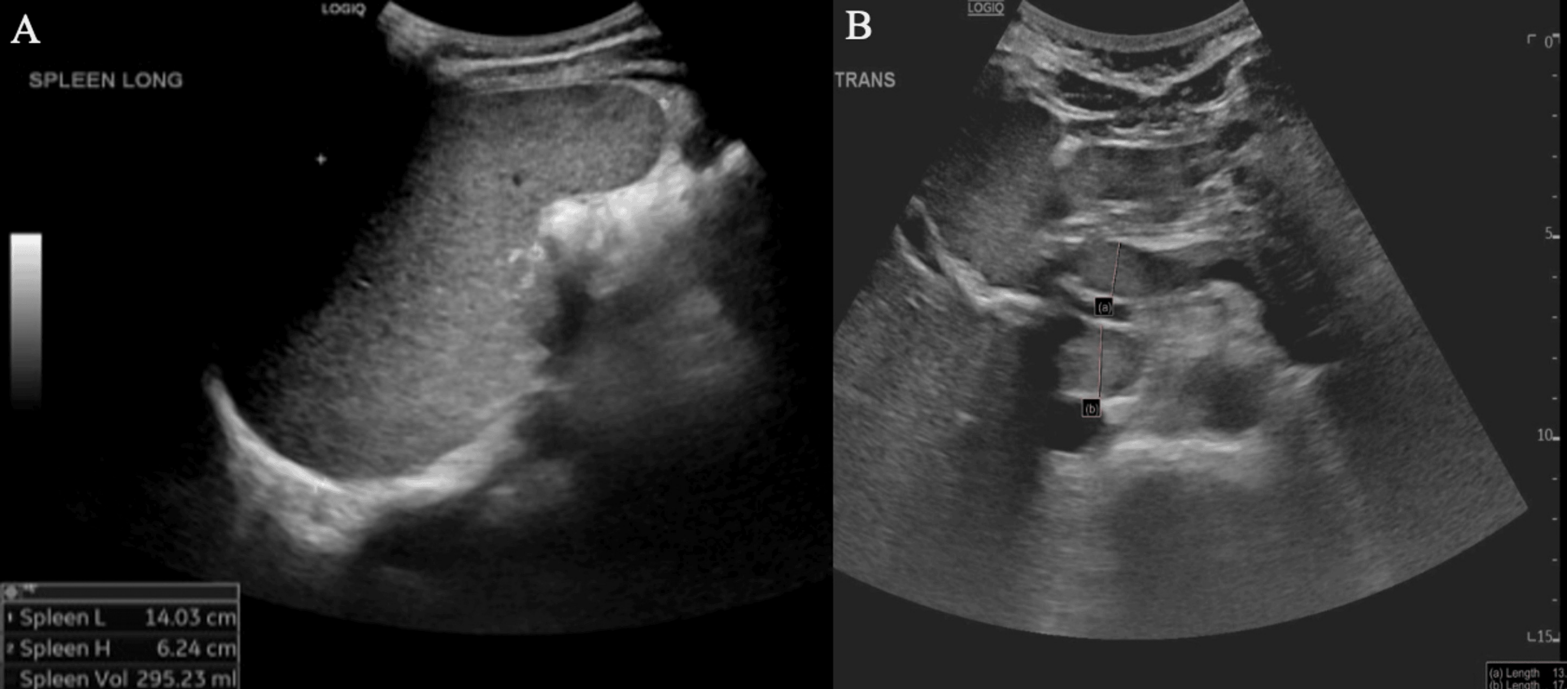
Abdominal Ultrasound (A) Abdominal ultrasound was remarkable for splenomegaly and periportal adenopathy. (B) The spleen measures 14.03 cm in length, with a volume of 295.23 mL. Periportal lymph nodes are seen, measuring up to 13 mm (node a) and 17 mm (node b) in the short axis.
